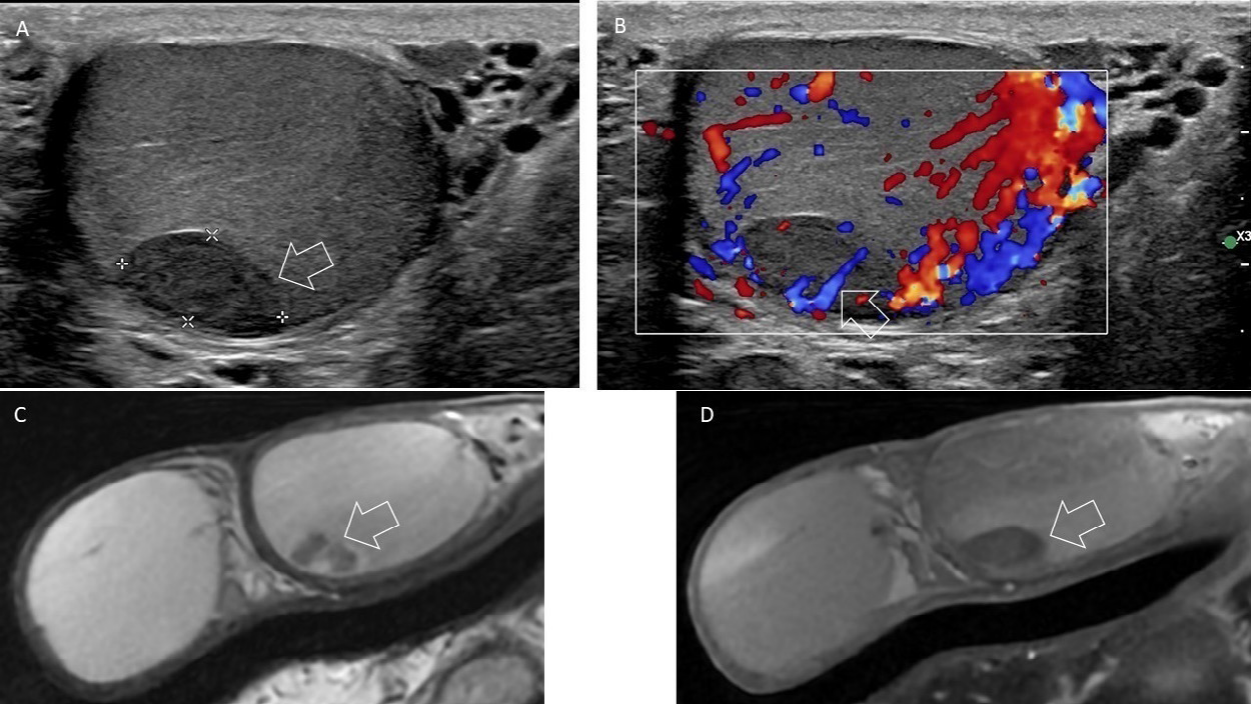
(A) Scrotal ultrasound showed a well‑defined hypoechoic lesion (arrow) (B) Color Doppler ultrasound showed no vascular distortion (arrow). (C) Axial T2‑weighted images showed a nodular hypointense lesion with hyperintense rim (arrow). (D) Post‑contrast T1‑weighted images showed no enhancement (arrow).
